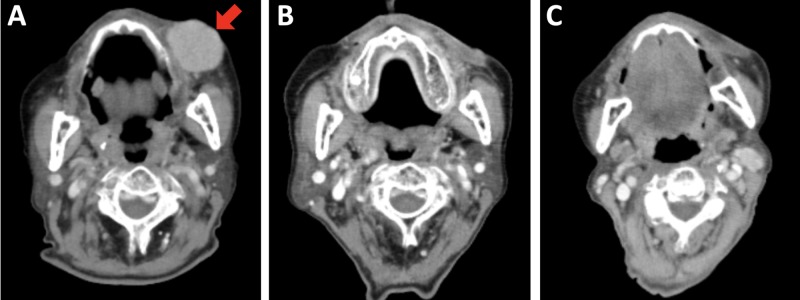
Computed tomography images of Merkel cell carcinoma. (A) Before initiation of radiation therapy. An arrow shows the tumor (36 mm in diameter) (B) In the middle of radiation therapy (i.e., at 36 Gy in 12 fractions). (C) Three months after initiation of radiation therapy.
